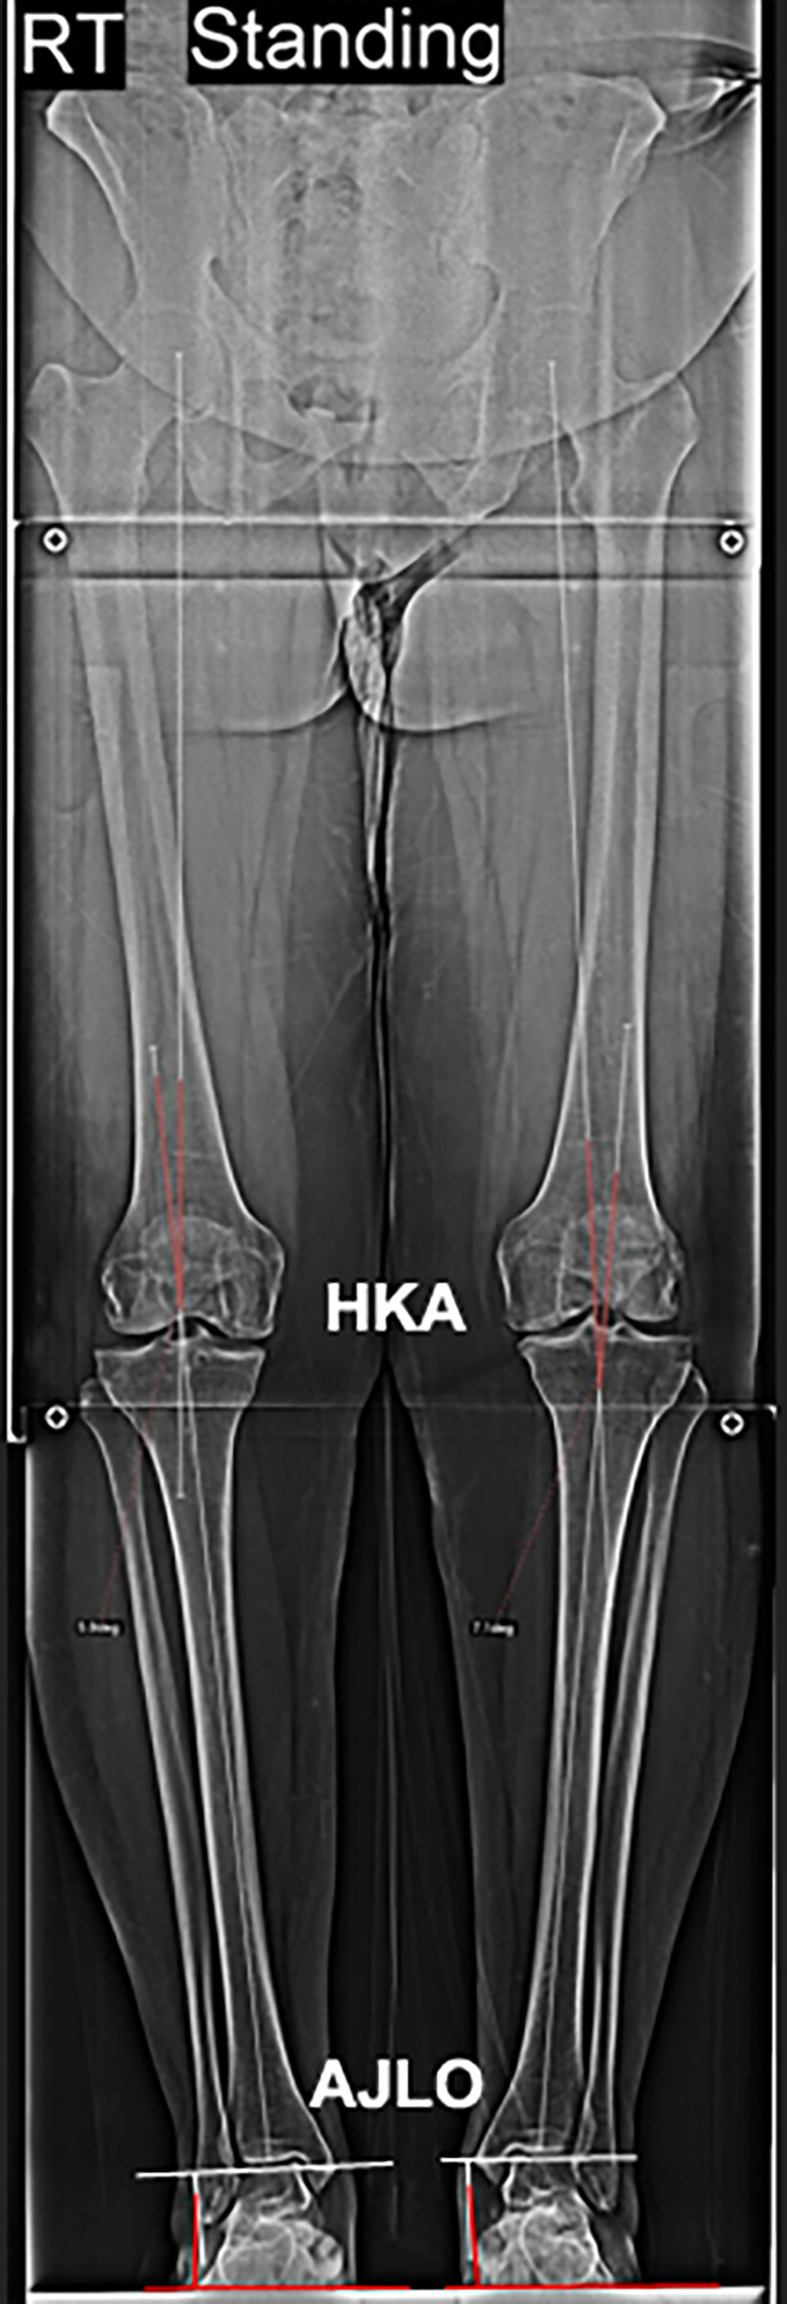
A standing whole lower limb radiograph showing Ankle joint line orientation (AJLO) and Hip-knee angle (HKA).
